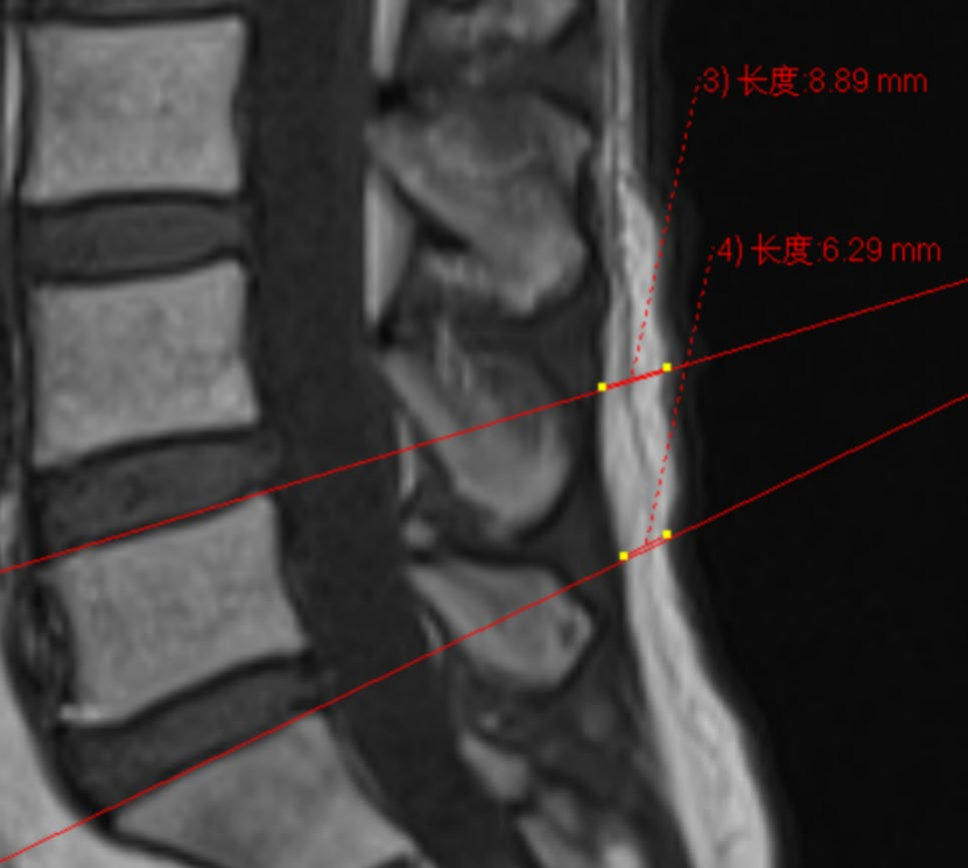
Mid-sagittal T1-weighted magnetic resonance image shows the measurement of lumbar subcutaneous fat tissue thickness in a Patient
